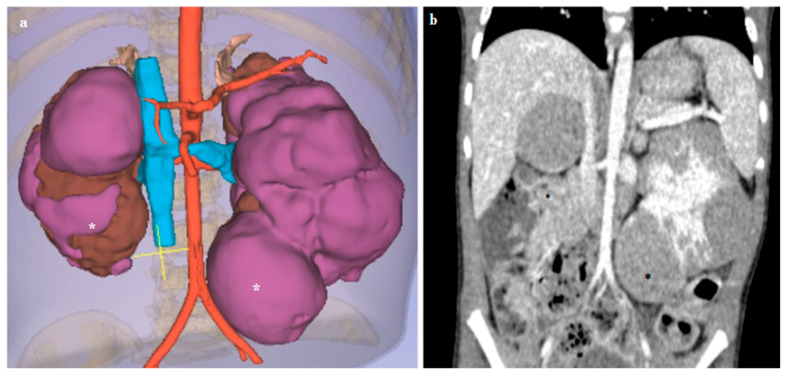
Virtual reality (VR) (a) and preoperative computed tomography (CT) (b) images of a 3-year-old girl with bilateral nephroblastomatosis (*). Both kidneys are in place, enlarged in size (right kidney 8 cm, left kidney 9 cm), with the parenchymal structure altered due to the presence of multiple solid lesions, the largest of which are located, respectively, at the upper pole of the right kidney, rounded in appearance, approximately 4 cm in diameter, and at the lower pole of the left kidney, approximately 4.5 cm in diameter. The lesions all have a nearly rounded morphology, and defined margins, albeit without a clear capsular structure.
